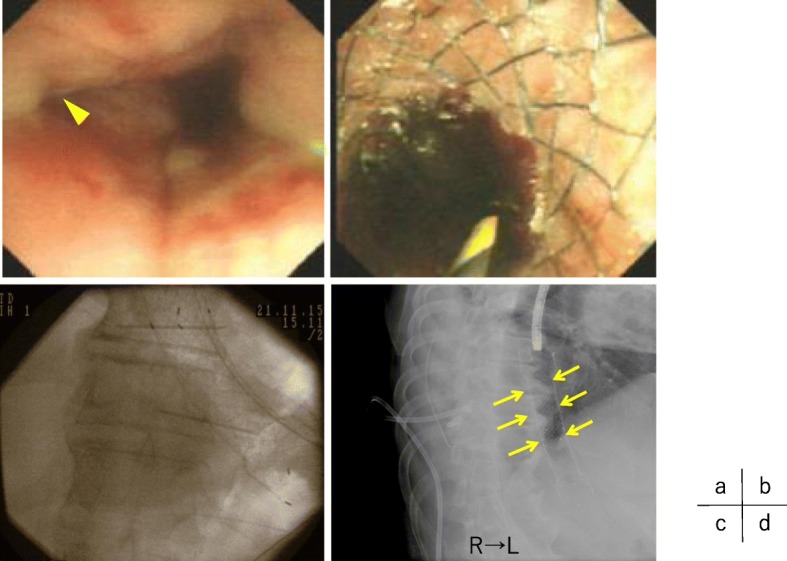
Esophageal stent placement. a Endoscopy revealed a pinhole perforation in the left posterolateral wall of the lower esophagus (arrowhead). b–d Esophageal stent placement (arrows) (b endoscopy, c fluoroscopy, and d chest X-ray)
